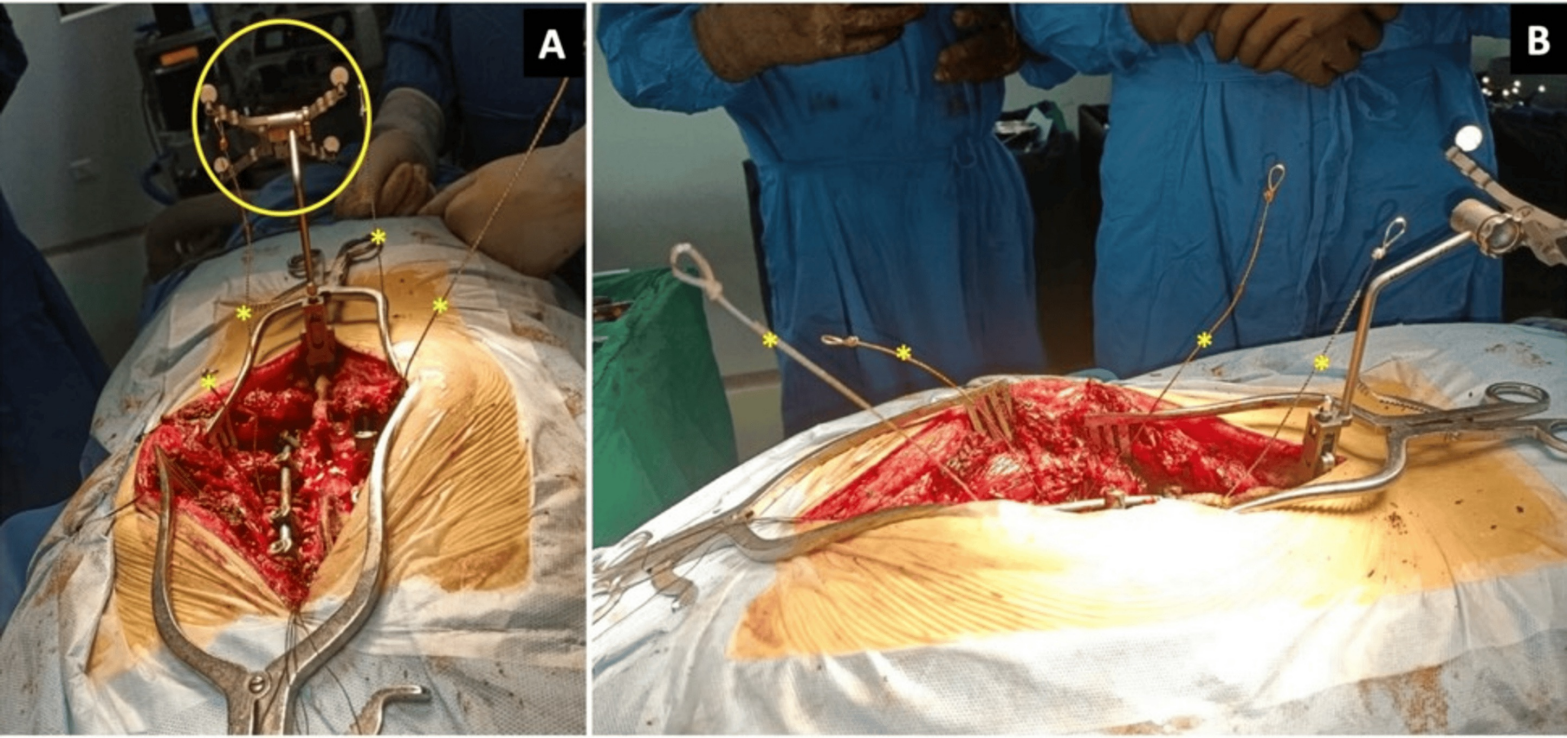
Intraoperative image taken just before the cranial and caudal cuts were made to free the T11 vertebra from the adjoining vertebrae. (A) The procedure guided by three-dimensional computed tomography navigation with the reference frame at the caudal end of the spinous process (yellow circle). A temporary stabilizing rod was inserted, and the Gigli’s saws were arranged to encircle the cranial and caudal disc of the involved T11 vertebra (the four ends of the two saw wires are marked with asterisks). (B) Lateral image showing the cranial and caudal arrangement of the two Gigli saw wires.
